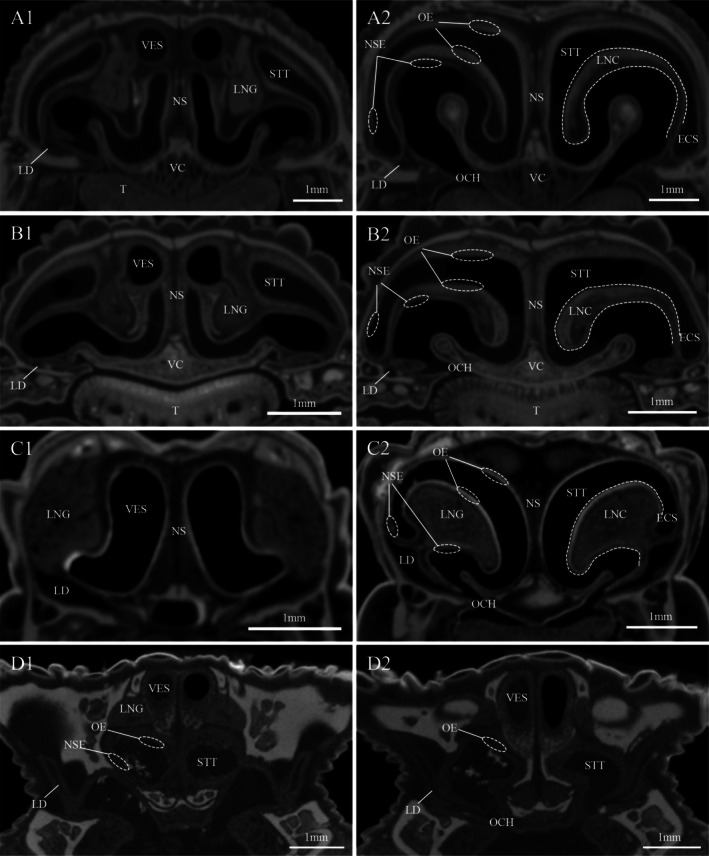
Transverse CT scans of the olfactory system of four species of lizards. (A1, A2) *T. roborowskii* (active foraging); (B1, B2) *T. dadunensis* (sit‐and‐wait foraging); (C1, C2) *E. roborowskii* (active foraging); (D1, D2) *P. axillaris* (sit‐and‐wait foraging). ECS, extra‐conchal space; LD, lacrimal duct; LNC, lateral nasal concha; LNG, lateral nasal gland; NSE, non‐sensory epithelium; OCH, outer choana; OE, olfactory epithelium; STT, Stammteil; T, tongue; VC, vomerine cushion; VES, vestibule.
